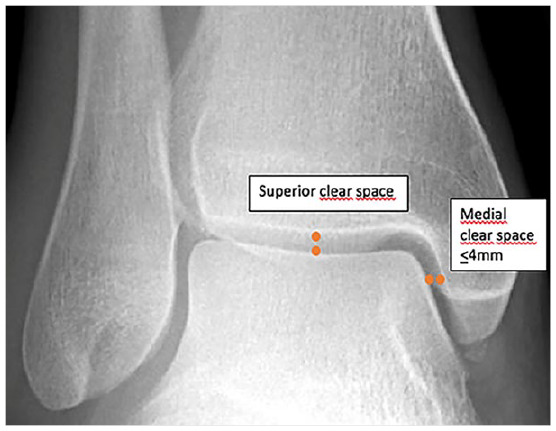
Ankle radiograph demonstrating measurements of medial clear space and superior clear space.
